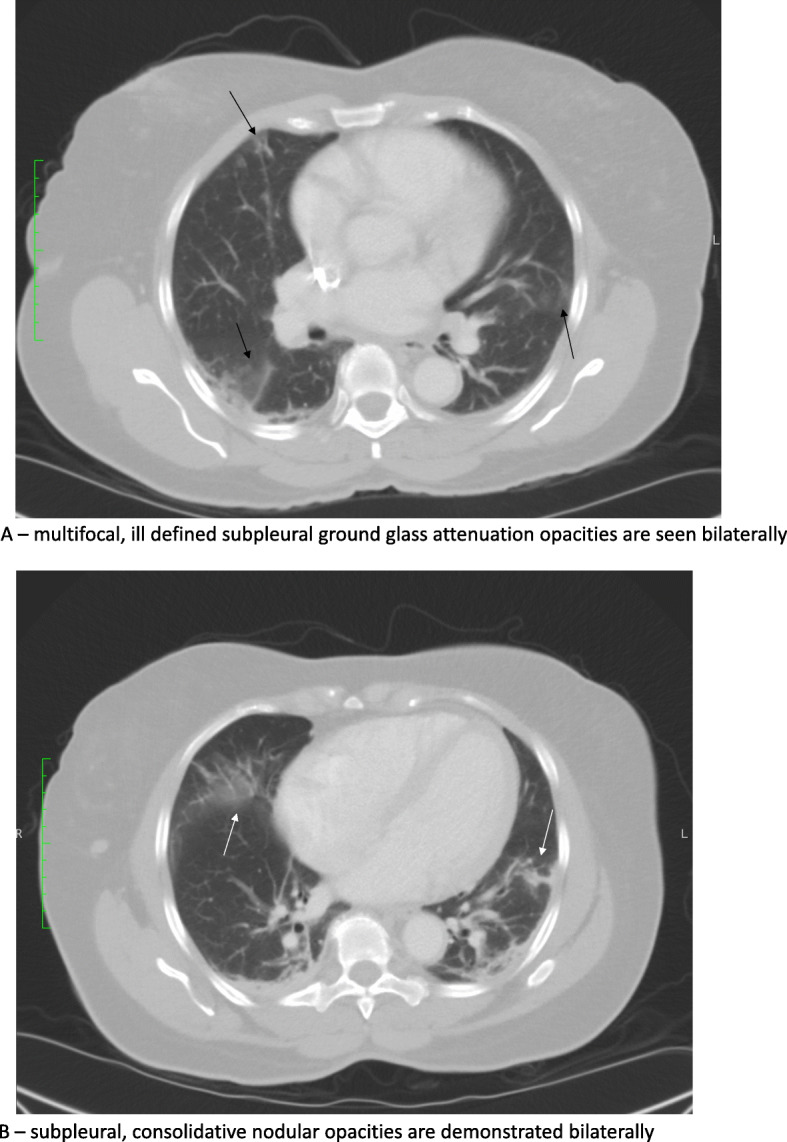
Contrast-enhanced chest computed tomography reveals multifocal, subpleural ground-glass attenuation opacities (arrow) (a) with nodular consolidations bilaterally (arrow) (b)
